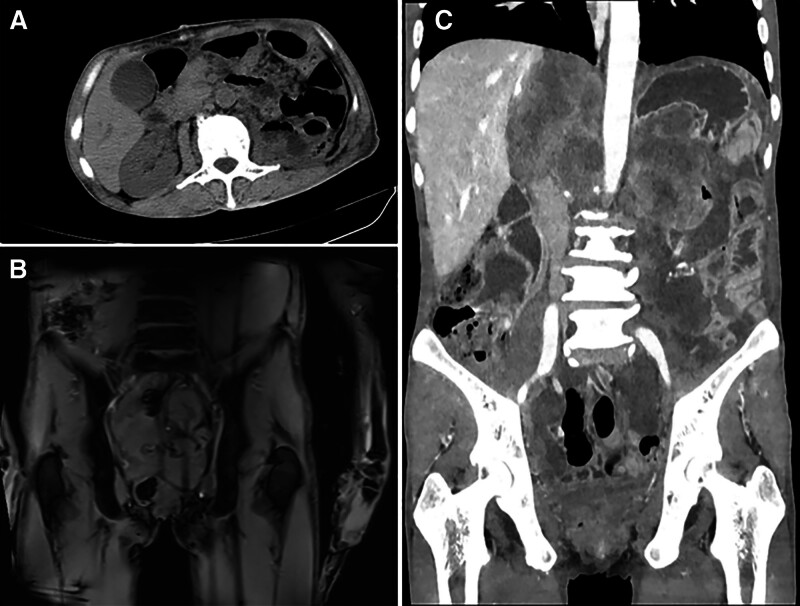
Abdominal imaging examination after the operation. (A) No obvious residual tumor was discovered in the retroperitoneal cavity. (B) MRI revealed multiple lesions in the pelvis. (C) CT showed multiple lesions in the abdomen.
